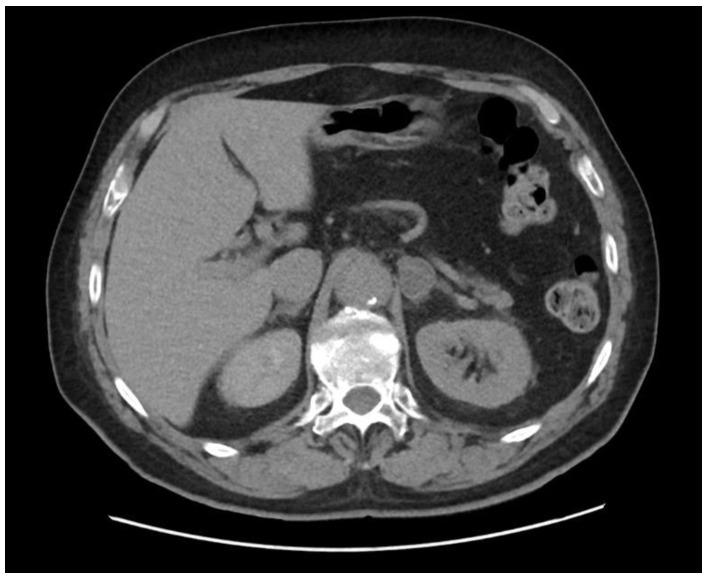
Non-contrast CT image showing AI.
